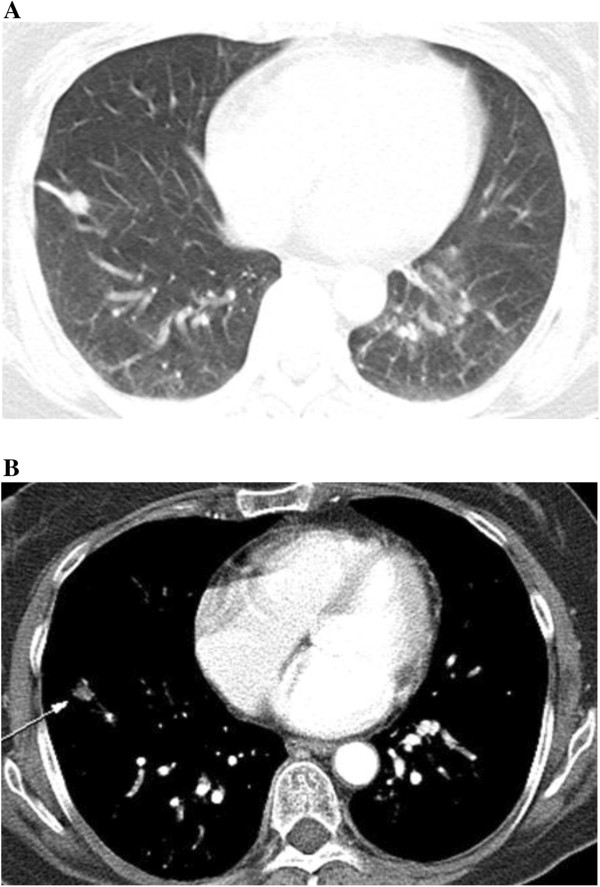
The F-18 fluorodeoxyglucose positron emission tomography-computed tomography (CT) scan shows a newly appeared pulmonary nodule in the right lower lung (A). CT scan shows a 9mm well-defined enhancing nodule in the anterobasal segment of the right lower lung (arrow) (B).
